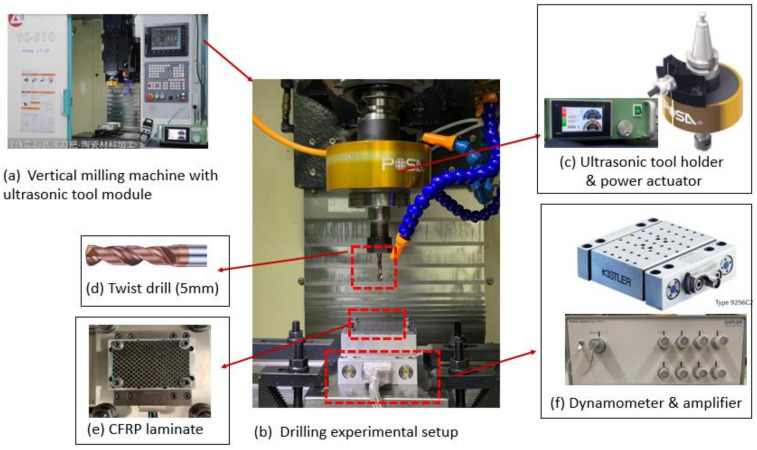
Experimental configuration: (a) vertical milling machine with ultrasonic tool module, (b) ultrasonic drilling experimental setup, (c) ultrasonic tool holder and power actuator, (d) twist drill, (e) CFRP workpiece, and (f) dynaometer with amplifier for measuring the cutting force.
